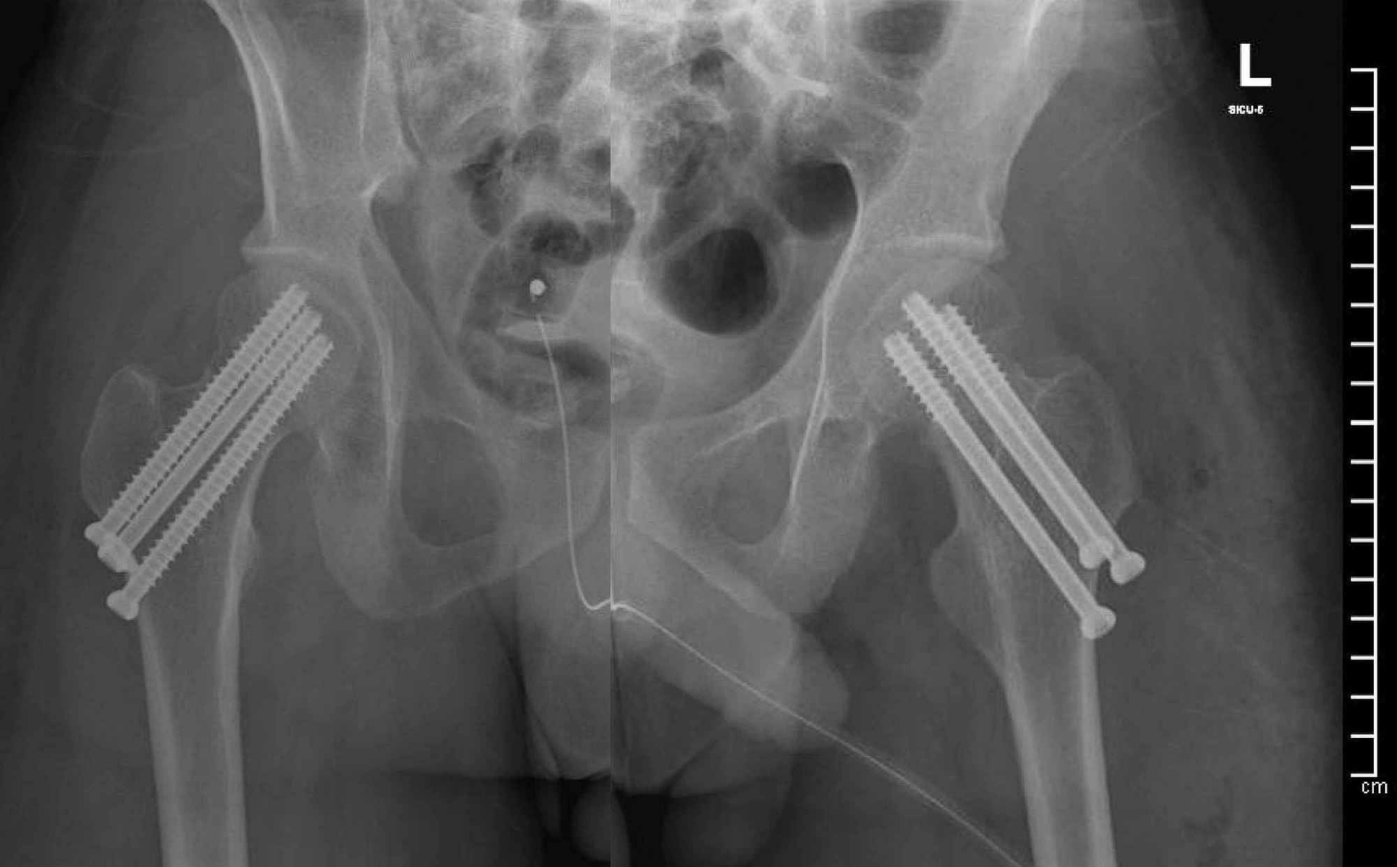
Pelvic X-ray after surgical fixation of femoral neck fractures.
